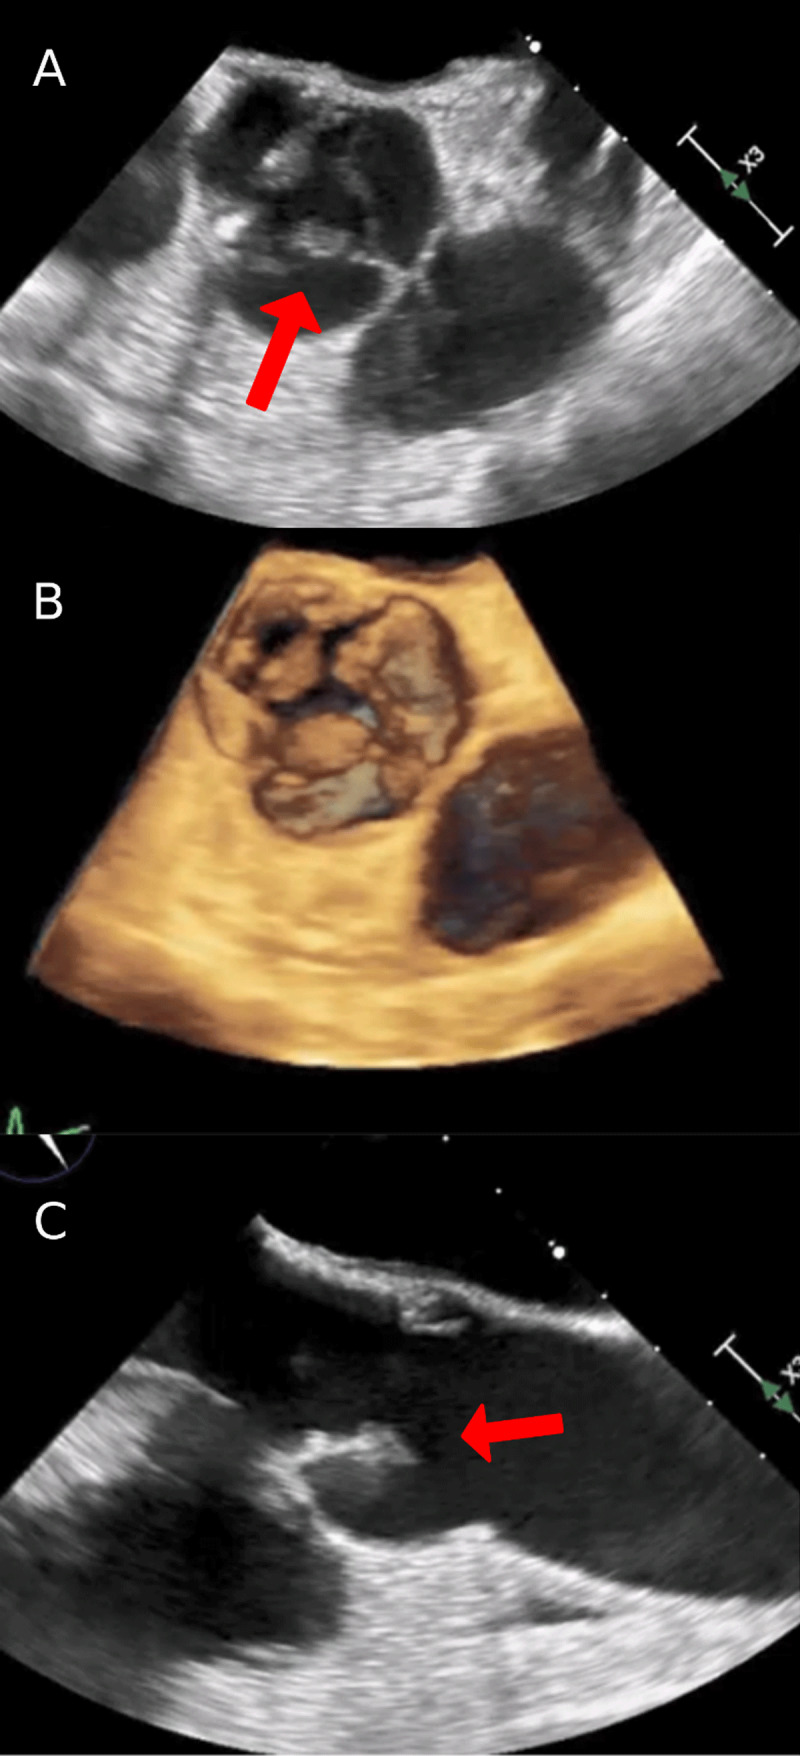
(A) Transesophageal echocardiogram (TEE) with short axis view of the aortic valve showing thickened non and right coronary cusp leaflet tips (arrow). (B) 3-dimensional image of TEE with short axis view of the aortic valve showing thickened non and right coronary cusp leaflet tips. (C) TEE with long axis view of the aortic valve showing thickened leaflet tips (arrow).
